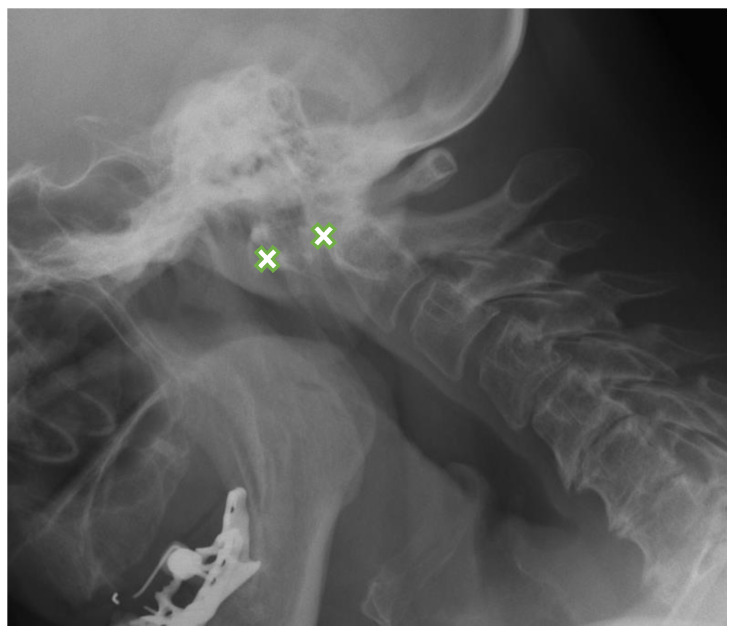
Lateral flexion view radiograph in a 63-year-old female with rheumatoid arthritis shows anterior AAS-8.8 mm (between crosses). AAS: atlanto-axial subluxation.
